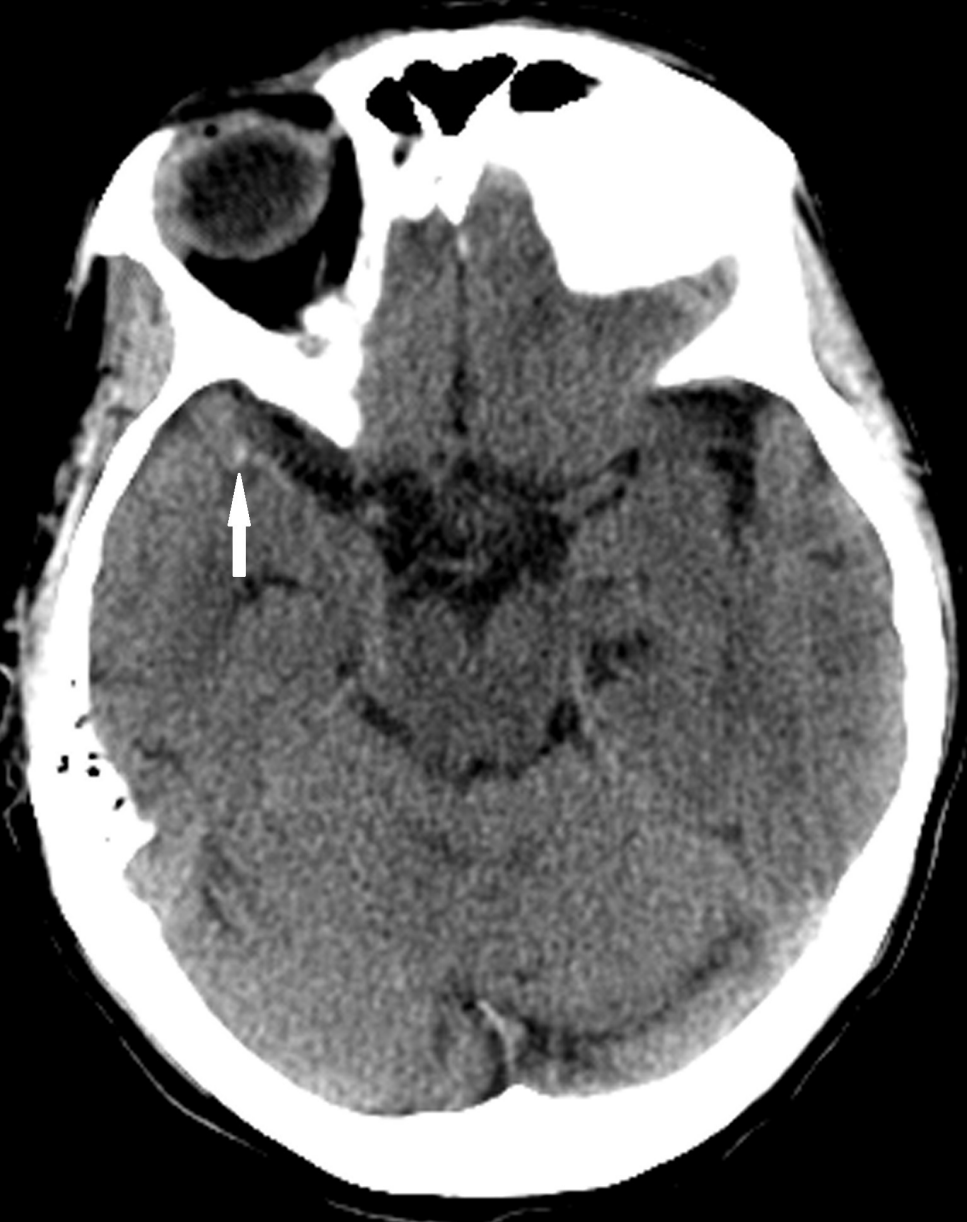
Noncontrast head computed tomography demonstrates markedly asymmetric density in the distal right middle cerebral artery (arrow) compatible with the dense middle cerebral artery sign
